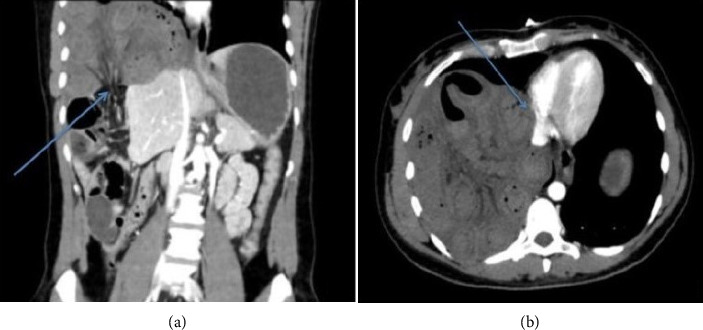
(a) Computed tomography, coronal section showing right diaphragmatic herniation (blue arrow). (b) Computed tomography, transverse section showing digestive handles in the right thorax cavity compressing the right-side heart chambers (blue arrow) (adapted from Deniau and Beloucif) [42].
